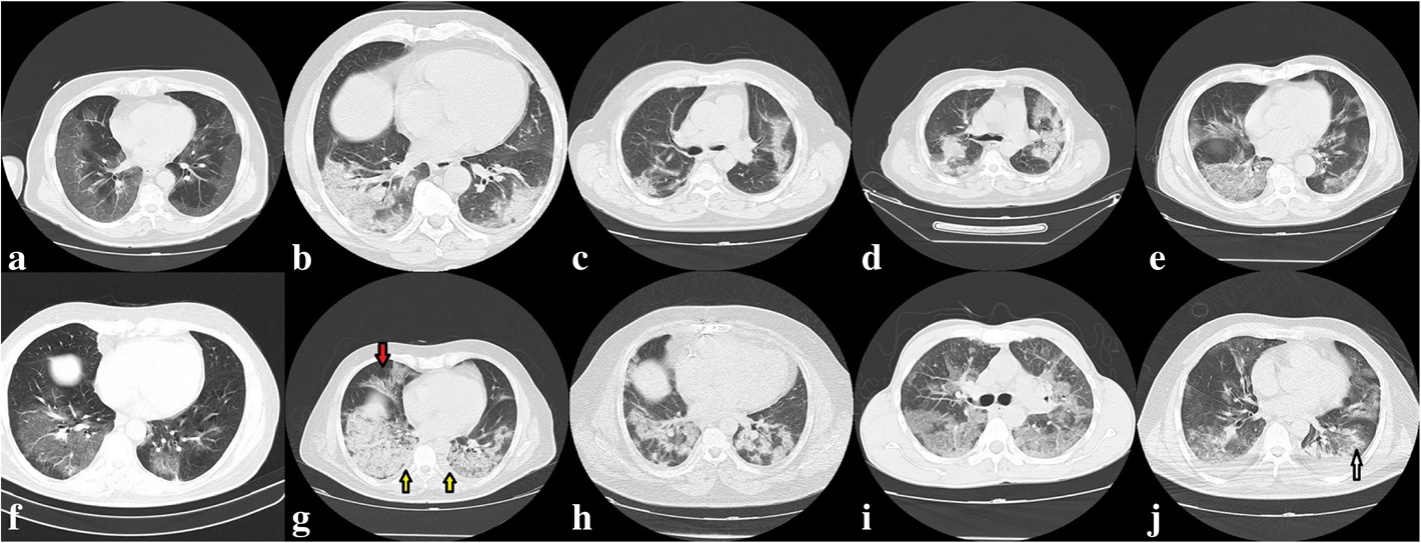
(a) Axial CT scans from an above 60 year-old male; Selected cut from non-contrast chest CT of lung window. Sub pleural crescent-shaped Ground-glass opacities as well as smooth interlobular septal thickening can be seen in both lungs, involving mostly peripheral zone; (b) Axial CT scans from an above 60 year-old male; selected image from non-contrast chest CT scans, lung window. Extensive consolidation with can be seen in both lower lobes with air bronchograms; (c) and (d) Axial CT scans from an above 50 year-old male at the level of carina; (C) Day 5 after symptom onset: patchy consolidation affecting the bilateral, peripheral lung parenchyma and (d) Day 7: expansion of consolidation in both lungs, as well as ground glass opacities in right side; (e) Axial CT scans from an above 60 year-old male; selected image from non-contrast chest CT scans, lung window. Mixed consolidation and ground glass opacities can be seen in both lower lobes, right middle lobe and lingula of left upper lobe; (f) and (g) Axial CT scans from an above 50 year-old male; selected image from non-contrast chest CT scans, lung window, (f) Day 3 after symptom onset: ground glass opacities in both lower lobe associated with mal focus of consolidation and (g) Day 7: expansion of consolidation in both lungs, as well as GGO in right middle lobe (black arrow); Mild pleural effusion is seen bilaterally (yellow arrows); (h) Axial CT scans from an above 50-year old female; selected image from non-contrast chest CT scans, lung window. Multiple patchy consolidation in both lower lobe; (i) Axial CT scans from an under 50 year-old male; selected image from non-contrast chest CT scans, lung window. Crazy-paving pattern (GGO with superimposed inter- and intralobular septal thickening) are seen bilaterally; (j) Axial CT scans from an above 50 year-old male; selected image from non-contrast chest CT scans, lung window. Ground-glass opacities affecting the bilateral lung field, reverse halo sign (ground-glass opacity surrounded by denser consolidation of crescentic shape) in left lower lobe (arrow), Pleural effusion is seen bilaterally
